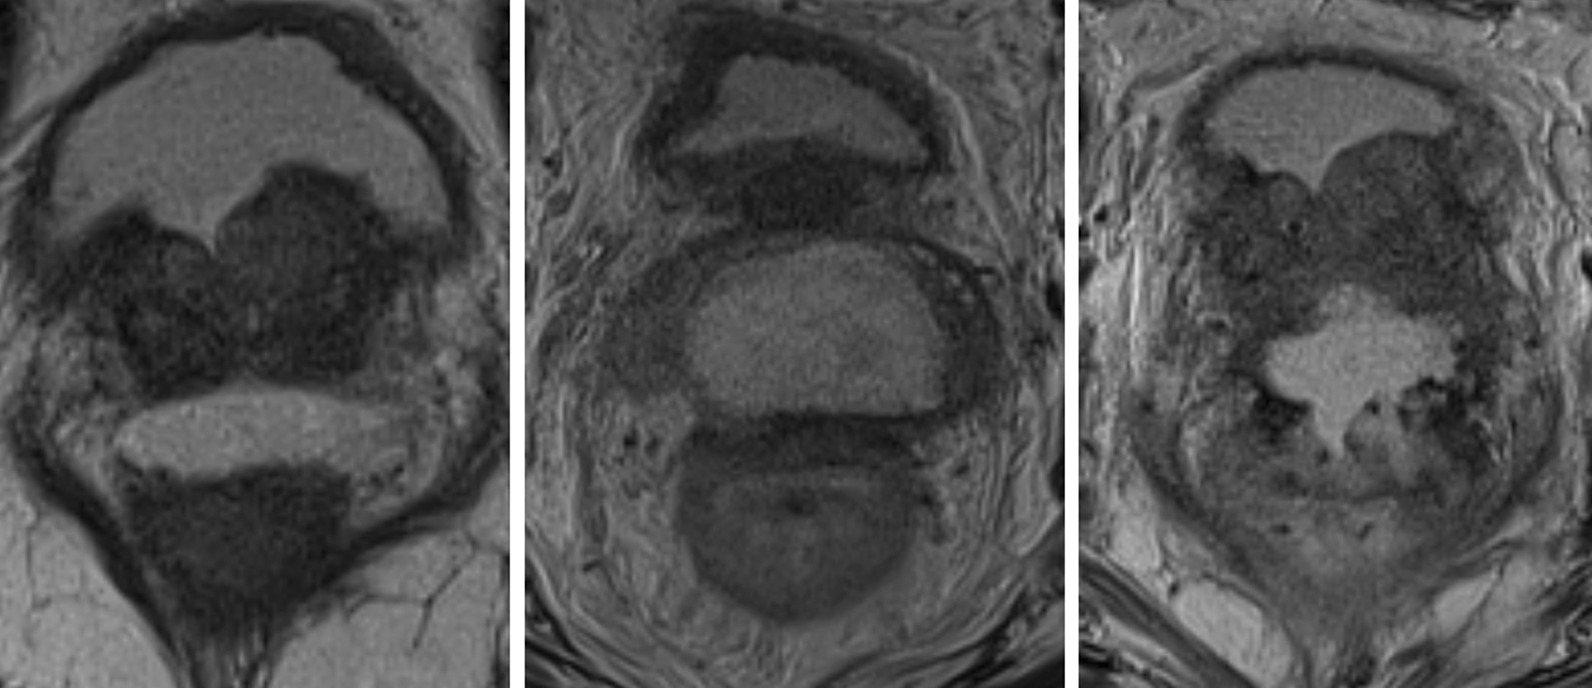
The changes of MRI findings after the SpaceOAR insertion. MRI taken 1 week after the SpaceOAR insertion (day 7, left). A peri-SpaceOAR abscess (day 61, middle) and a rectal perforation were detected on MRI (day 120, right)
